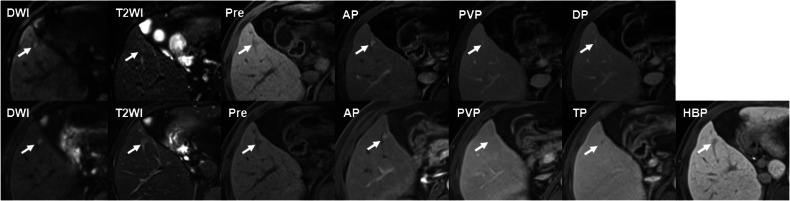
A 54-year-old man with subcentimeter recurrent HCC (0.7 cm, white arrow) underwent both ECA-MRI and EOB-MRI within 8 days. On ECA-MRI, this lesion showed T2WI mild-moderate hyperintensity and restricted diffusion, without nonperipheral washout on PVP or DP, and thus was not diagnosed as recurrent HCC based on ECA-MRI. In EOB-MRI conducted 8 days later, this lesion showed T2WI mild-moderate hyperintensity, restricted diffusion and nonperipheral washout on PVP or TP, thus being diagnosed as recurrent HCC on EOB-MRI-based modified diagnostic algorithm. EOB-MRI, gadoxetate acid-enhanced MRI; ECA-MRI, extracellular contrast agent-enhanced MRI; AP, arterial phase; PVP, portal venous phase; DP, delayed phase; TP, transitional phase; HBP, hepatobiliary phase
